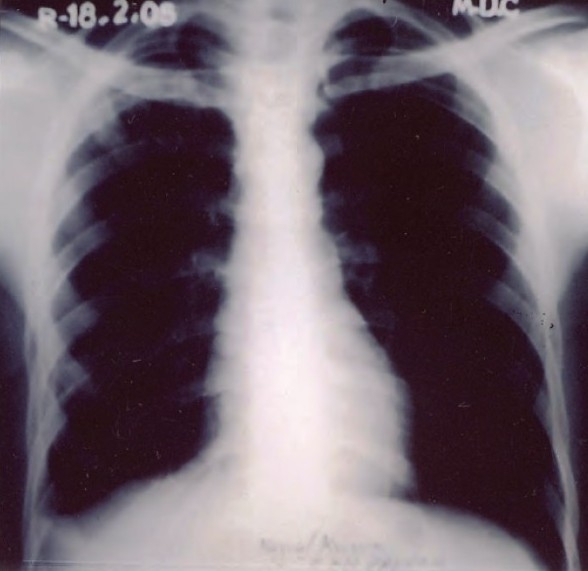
Xray chest PA view at 4 months showed clearing of the pleural on the left side with blunting of right costo-phrenic angle due to thickening of the pleura.
